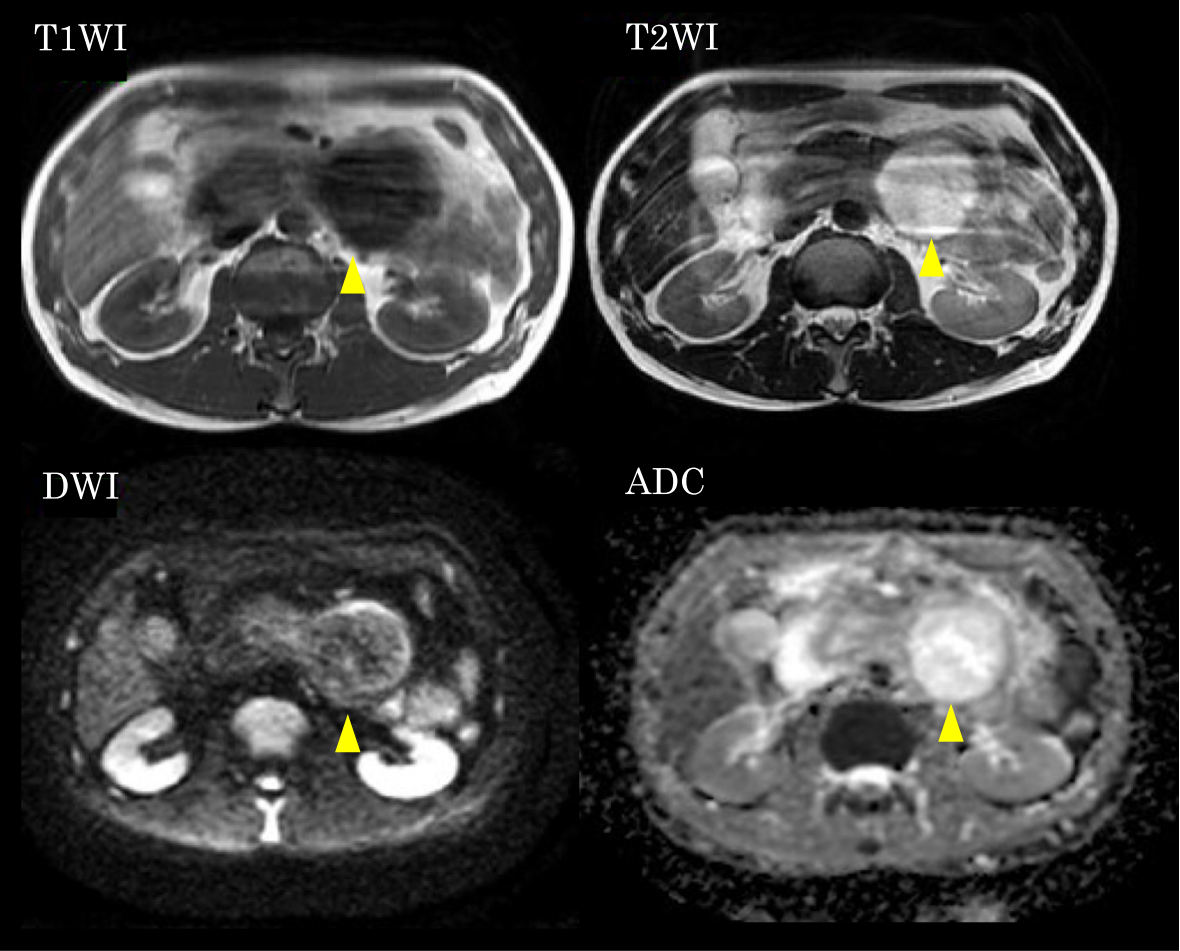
MRI showed T1 WI low, T2 WI high, ADC high, DWI high mass (arrow)
